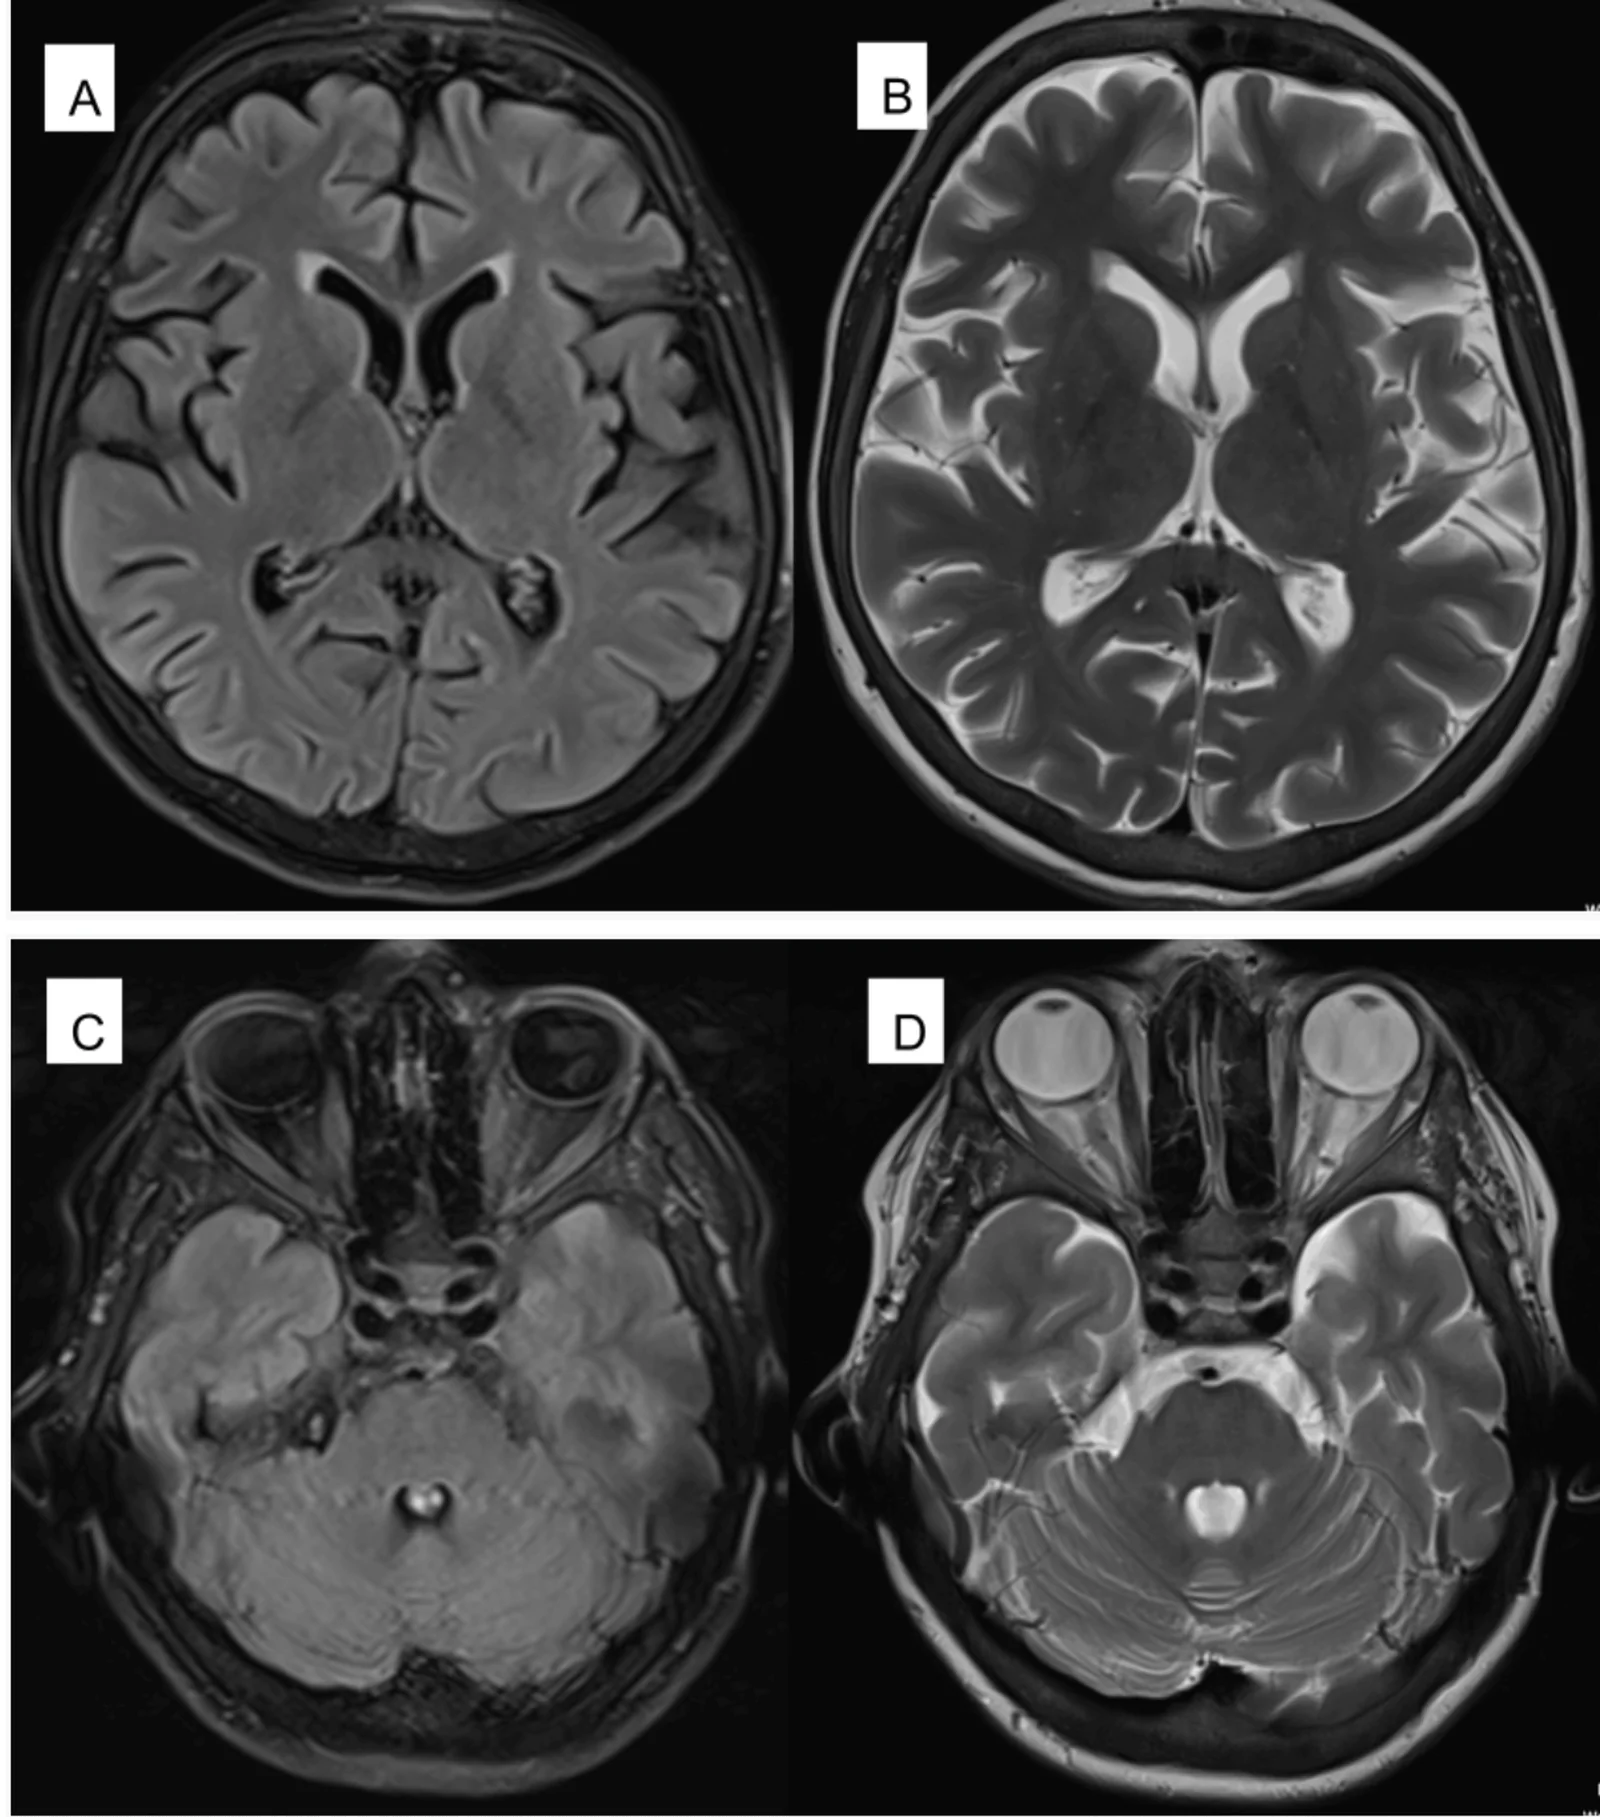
Brain MRI on day 60 after onset Axial fluid-attenuated inversion recovery (FLAIR) and axial T2-weighted hemisphere (A, B) images, as well as axial FLAIR and T2-weighted posterior fossa images (C, D), show near-resolution of the lesions.
